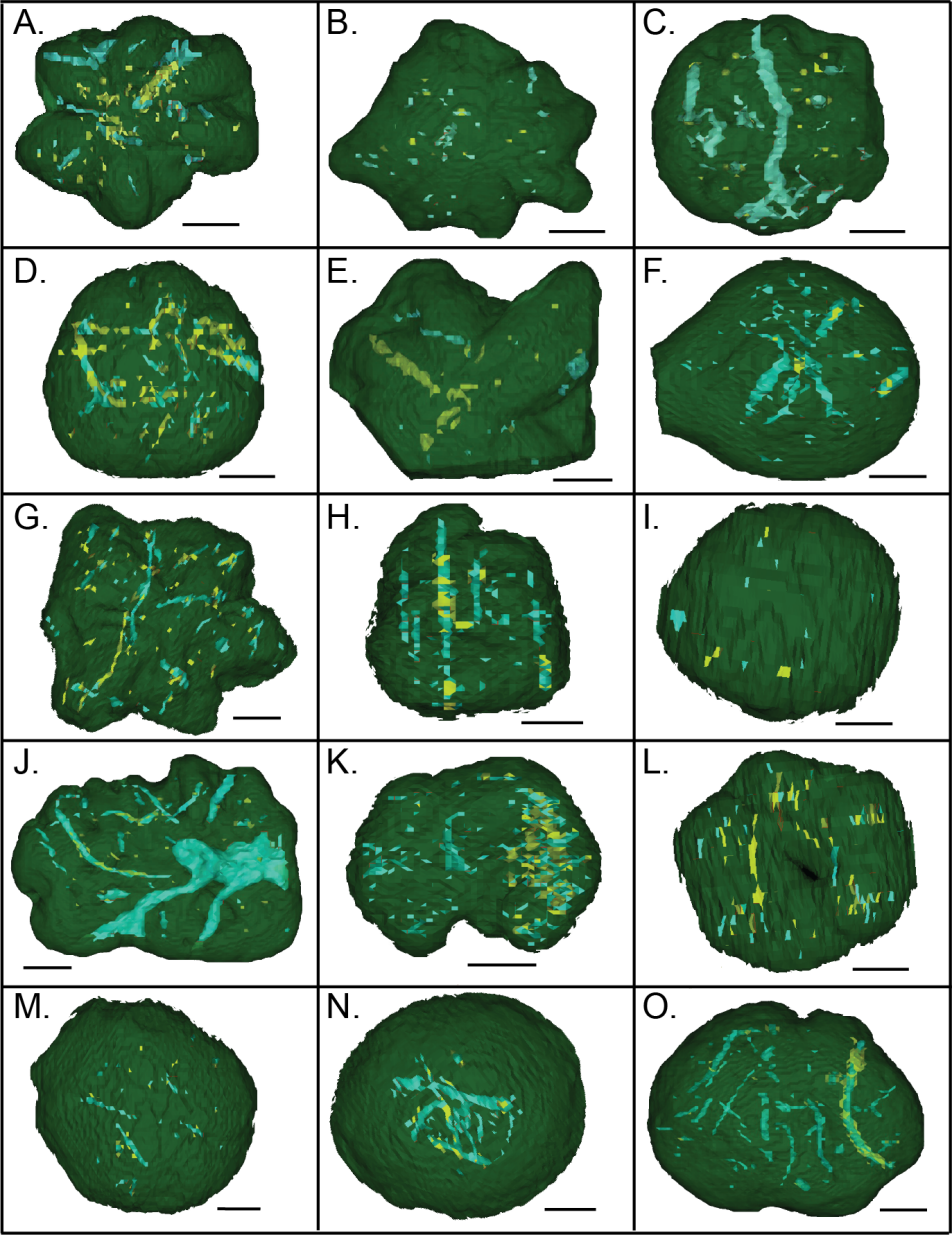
CT scans of *Brooksella* viewed from the top surface (A–L) and concretions (M–O). Green indicates external morphology, blue indicates low density mineral phases and voids, and yellow indicates higher density mineral phases. Scale bar = one cm. (A–L); figured *Brooksella* samples: (A) UGA 1; (B) UGA 3; (C) UGA 6; (D) UGA WSL2.AL1; (E) 55; (F) UGA LSV1.AL2; (G) UGA WSL2.AL2; (H) UGA 98; (I) UGA WSL2.AL12; (J) UGA 17; (K) UGA 155; (L) UGA WSL2.AL21; figured concretion samples: (M) UGA 103; (N) UGA 56; (O) UGA 60.
